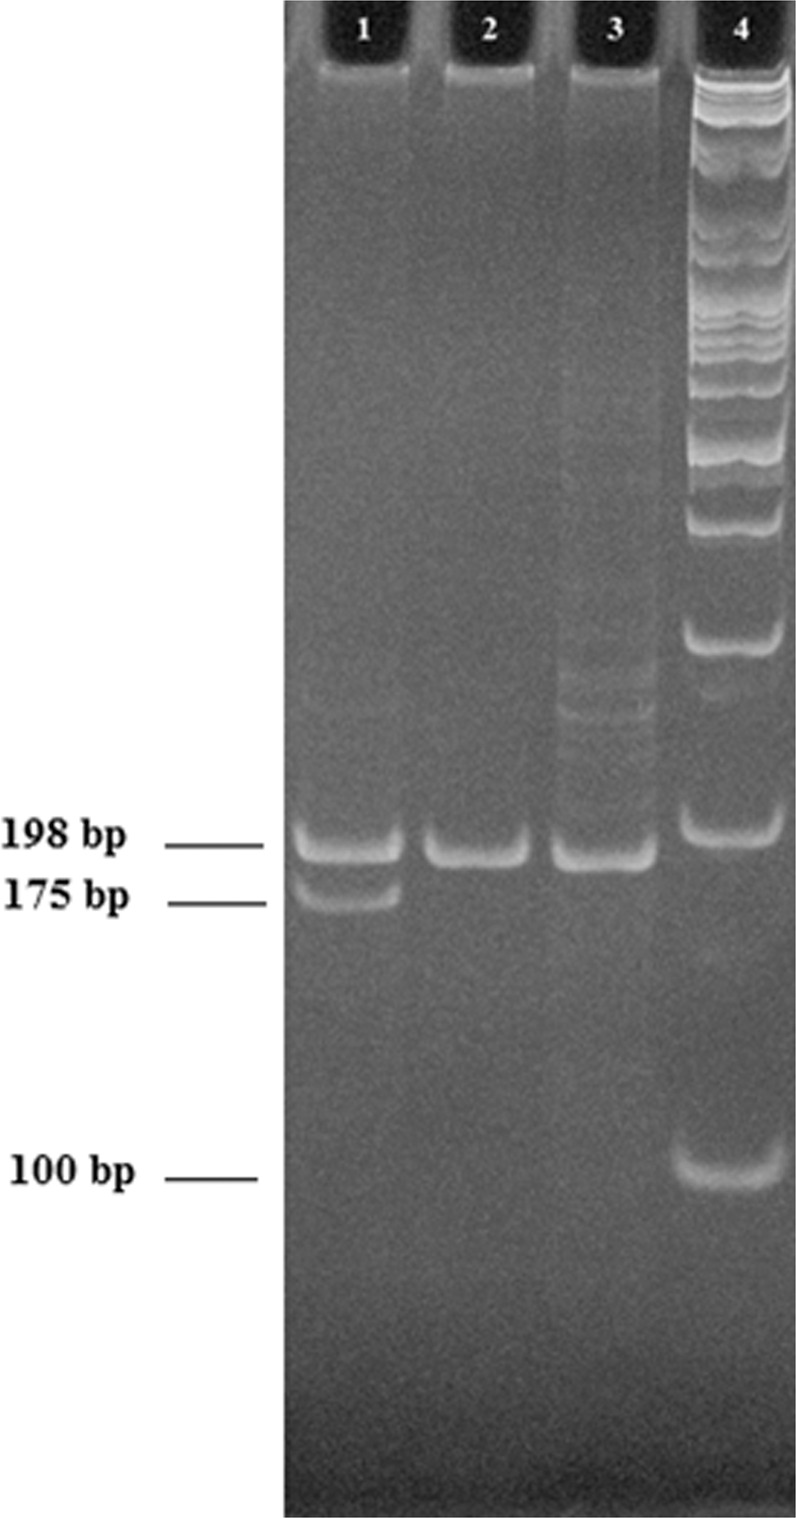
Genotypes of MTHFR C677T polymorphism on 10% polyacrylamide gel electrophoresis showing PCR-RFLP products. L1: CT genotype; L2: CC genotype; L3: undigested PCR product; L4: DNA standard marker (100 bp).
